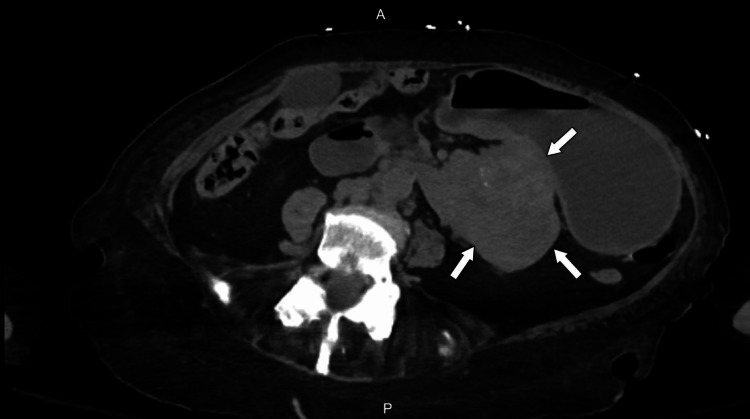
Abdominal computed tomography (CT) scan showing left retroperitoneal/duodenal mass (arrows). A-anterior, P-posterior.
